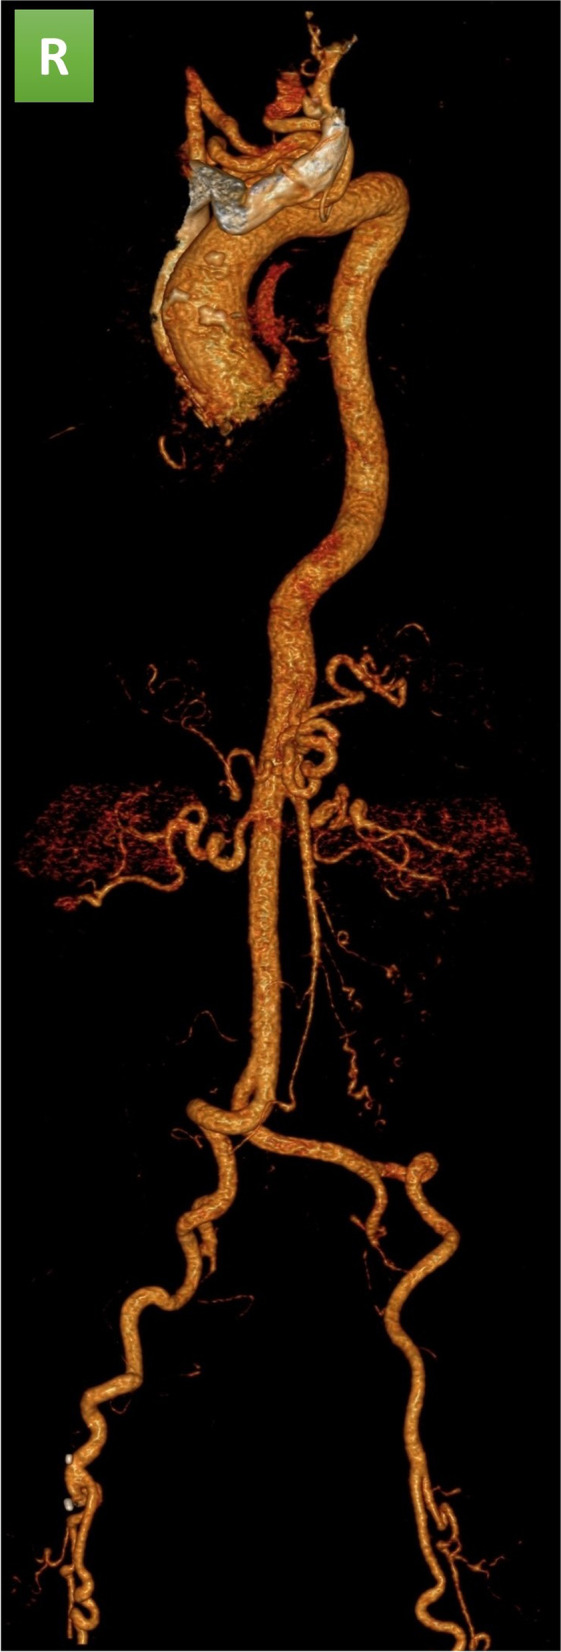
Three-dimension reconstruction of Angio-CT scan of the thoracic and abdominal aorta of the ATS patient, showing tortuosity of thoracic and abdominal vessel without stenosis. R: right
